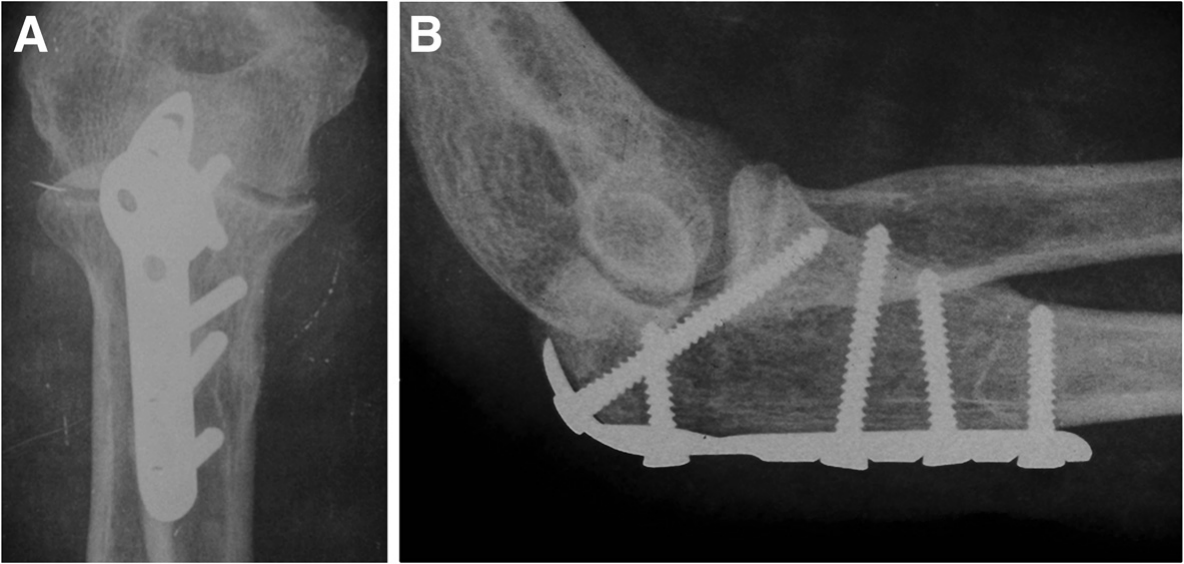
Postoperative radiographic assessment demonstrated nearly anatomical reduction of the olecranon fractures. (A, the anteroposterior view. B, the lateral view).
